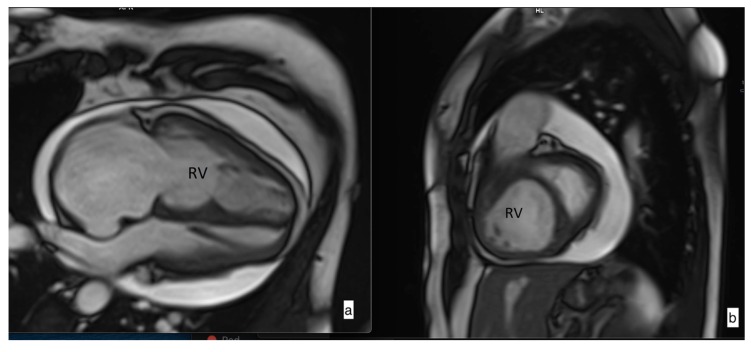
Steady-state free precession (SSFP) cine imaging by cardiac magnetic resonance imaging of a patient with pulmonary arterial hypertension. SSFP in the four-chamber (a) and short-axis (b) orientation at early diastole showing right heart chamber dilatation and leftward ventricular septal bowing due to increased pressure in the right ventricle (RV). Pericardial effusion is also noticed.
